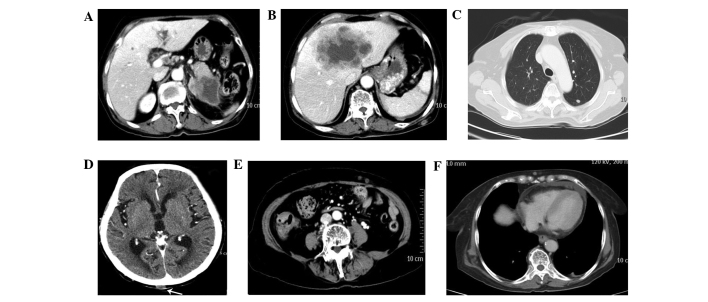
(A) Computed tomography (CT) scan revealed an enlarged pancreatic tail with a low-density soft tissue mass measuring 7 cm in diameter with (B) hepatic focal lesions and (C) lung lesions. (D) Cutaneous metastasis is present on the CT scan at the occipital scalp, (E) right upper chest and (F) left abdomen.
